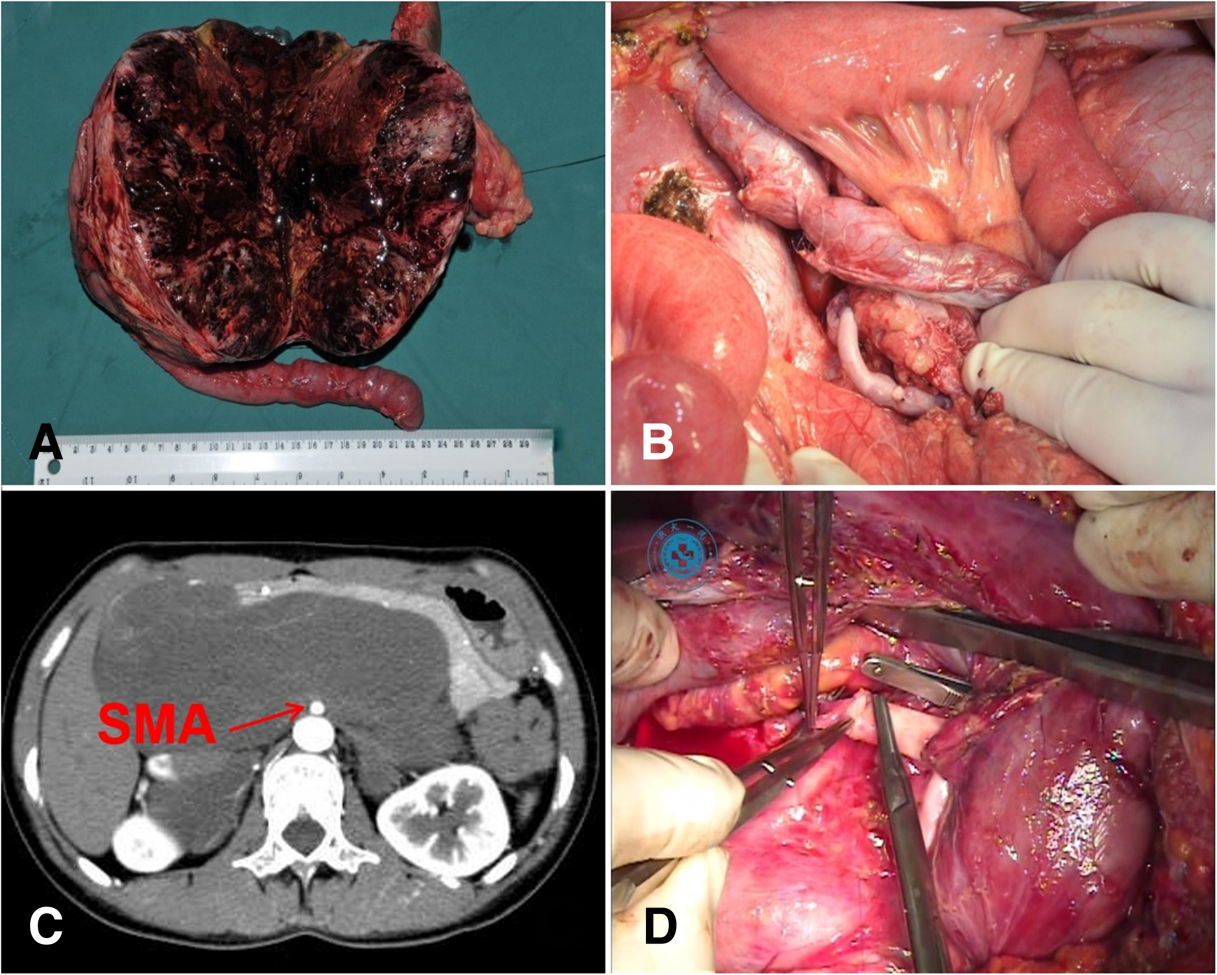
a A giant solid pseudopaillary tumor (16 cm) located in the pancreatic head and involving the superior mesenteric artery (SMA). b SMA reconstruction using an autologous great saphenous vein. c CT scan of a retroperitoneal ganglioneuroma involving the pancreatic head and SMA. d SMA reconstruction using a size-matched allogeneic frozen iliac artery graft
